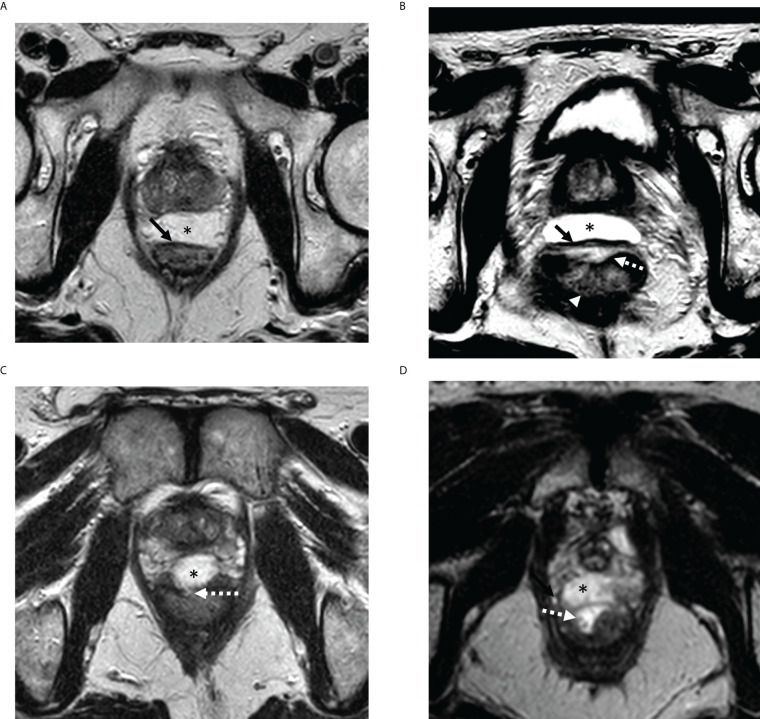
Representative MRI examples of rectal wall infiltration (RWI) scoring system on axial T2-weighted imaging. (A) RWI score 0: Hydrogel (*) is well circumscribed and outer wall of rectum is smooth (solid arrow) with no signal changes. (B) RWI score 1: Edema only appreciated in the anterior rectal wall (broken arrow) but outer rectal wall is smooth without breach (solid arrow). Note lack of edema in the posterior rectal wall (arrowhead) (C) RWI score 2 (Superficial RWI): Focal breach (broken arrow) is noted in outer rectal wall contiguous with the hydrogel (*). (D) RWI score 3 (Deep RWI): Hydrogel (*) extends to inner rectal wall and lumen (broken arrow). RWI, rectal wall infiltration.
